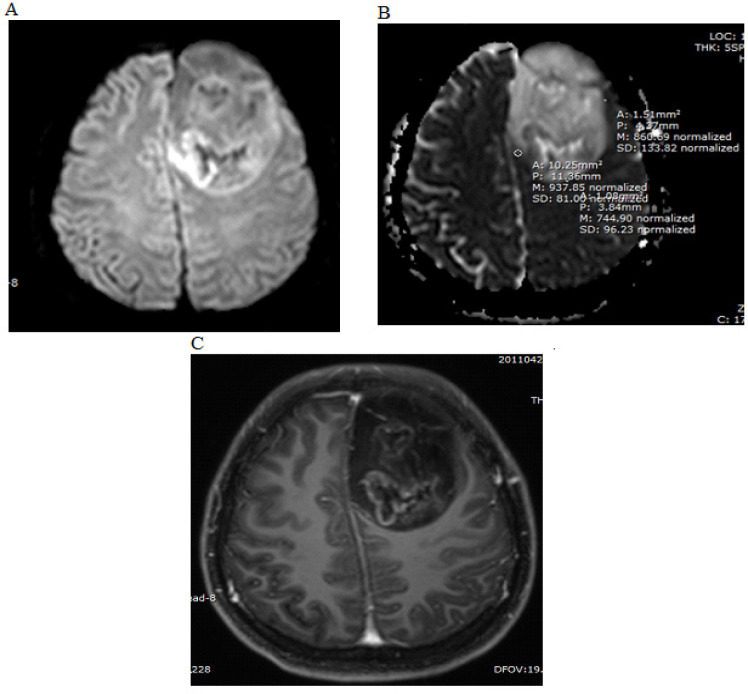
The Same Patient. DWI (A), ADC Map (B) and Axial Post Contrast T1WI (C) Demonstrated Restricted Diffusion Areas at Perienhancing Area. At this image, three ROIs were drawn on the ADC map (B) at the area with minimal ADC value. The measured ADC values in this image were 0.937, 0.744 and 0.86 x10^-3^mm^2^/s. The mean ADC value of the perienhancing area of this case was 0.86 x10^-3^mm^2^/s. Post-contrast image (C) showed that no enhancing part was included in the measurement of perienhancing area
